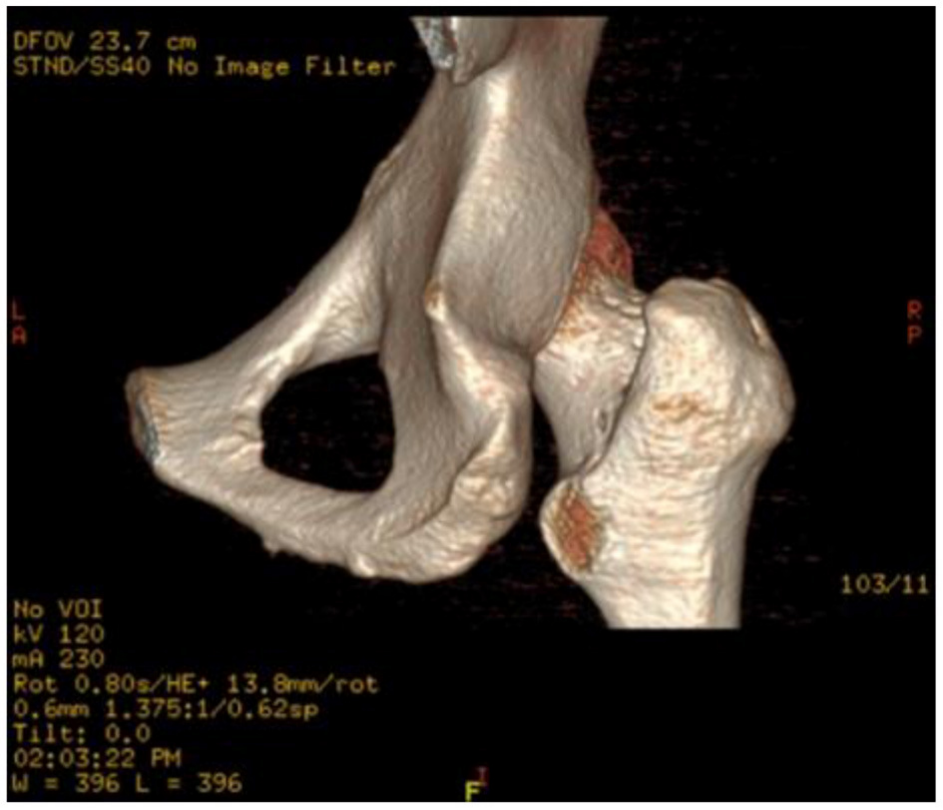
Postoperative three-dimensional CT of the affected hip. Approximately one-third of the posterior medial lesser trochanter was resected.
